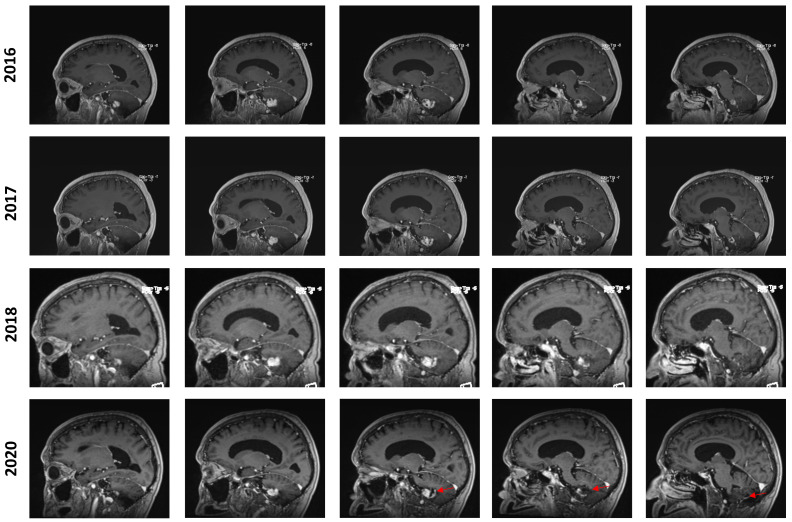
Longitudinal follow-up. Representative capture of sagittal T1-weighted MRI control imaging. The date of imaging is represented. Images are organized left to right and represent a left–right progression in the sagittal plane. The red marker highlights the appearance of an enlarging cystic component in the posterior aspect of the lesion in 2020.
